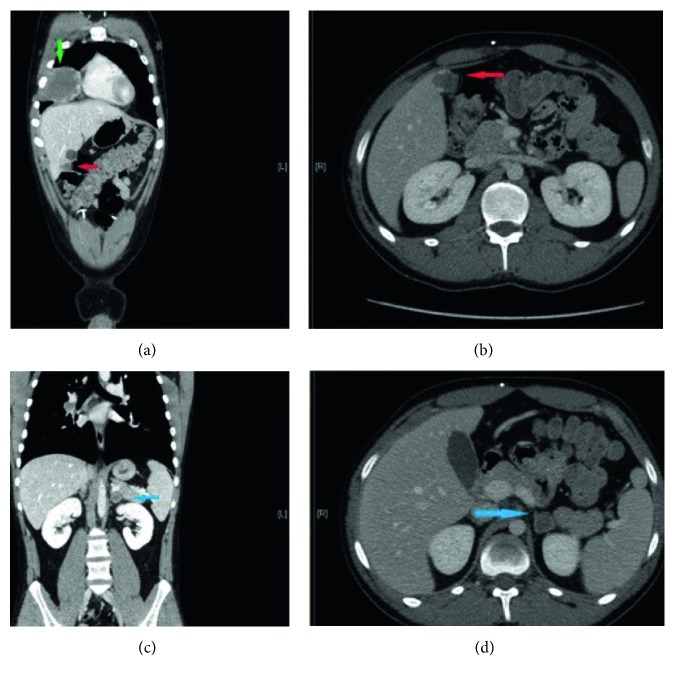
CT scan of the thorax and abdomen showing the lung (green arrow), liver (red arrow), and left adrenal (blue arrow) lesions.
